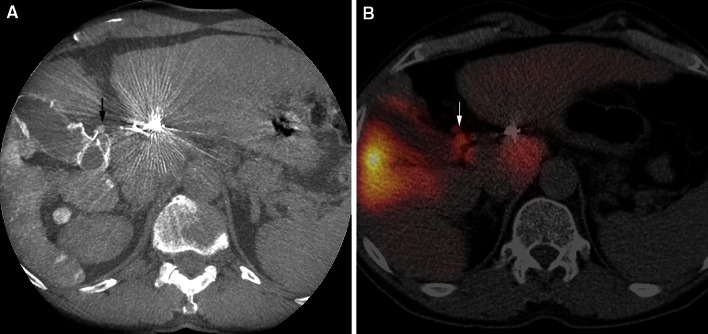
Comparison of C-arm CT and SPECT/CT in a patient with extrahepatic shunting. A C-arm CT shows extrahepatic shunting in the duodenal region (*black arrow*), caused by a collateral branch from the cystic artery. B Corresponding extrahepatic ^99m^Tc-MAA activity in the duodenal region on SPECT/CT (*white arrow*)
